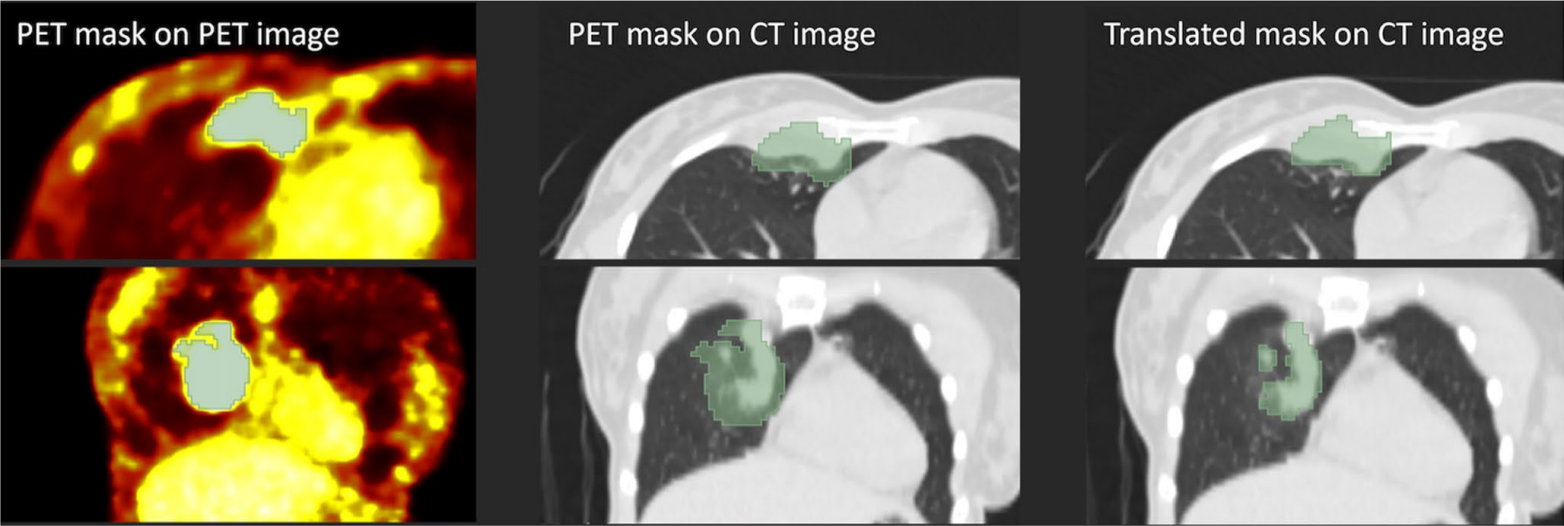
Segmentation of the PET and the CT images. The first row shows axial, and the second shows coronal slices. Left: PET image with the original mask. Middle: CT image with the upsampled PET mask (Case 1). The tumor is not adequately covered, potentially affecting the extracted radiomics features from the segmented area. Right: CT image with the upsampled and translated mask (Case 2) shows a better fit to the tumor after the transformation
